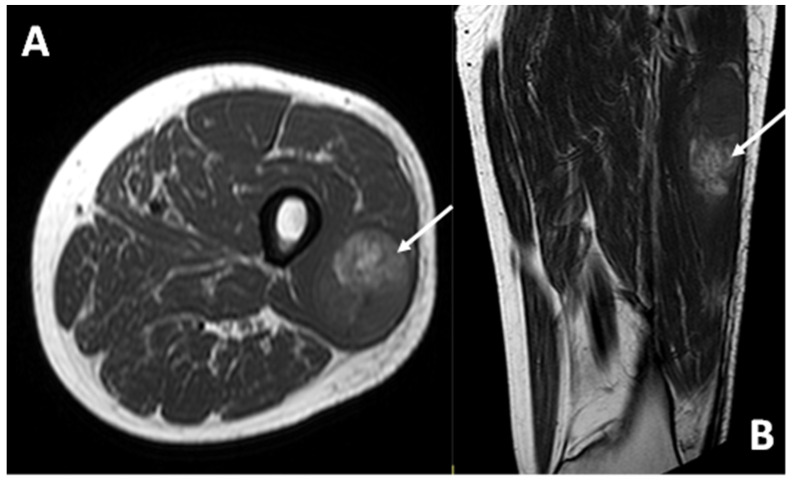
T1-weighted sequences in axial (A) and coronal (B) planes of a deep-seated CCS of the thigh: note the high signal intensity of the lesion (arrows) compared to the normal adjacent muscle, the deep location, and the smooth margins.
